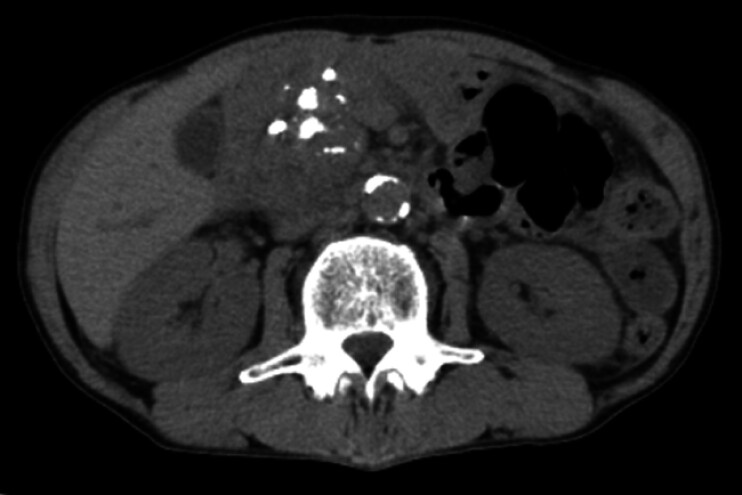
Computed tomography image showing huge pancreatic stones in the main pancreatic duct.
